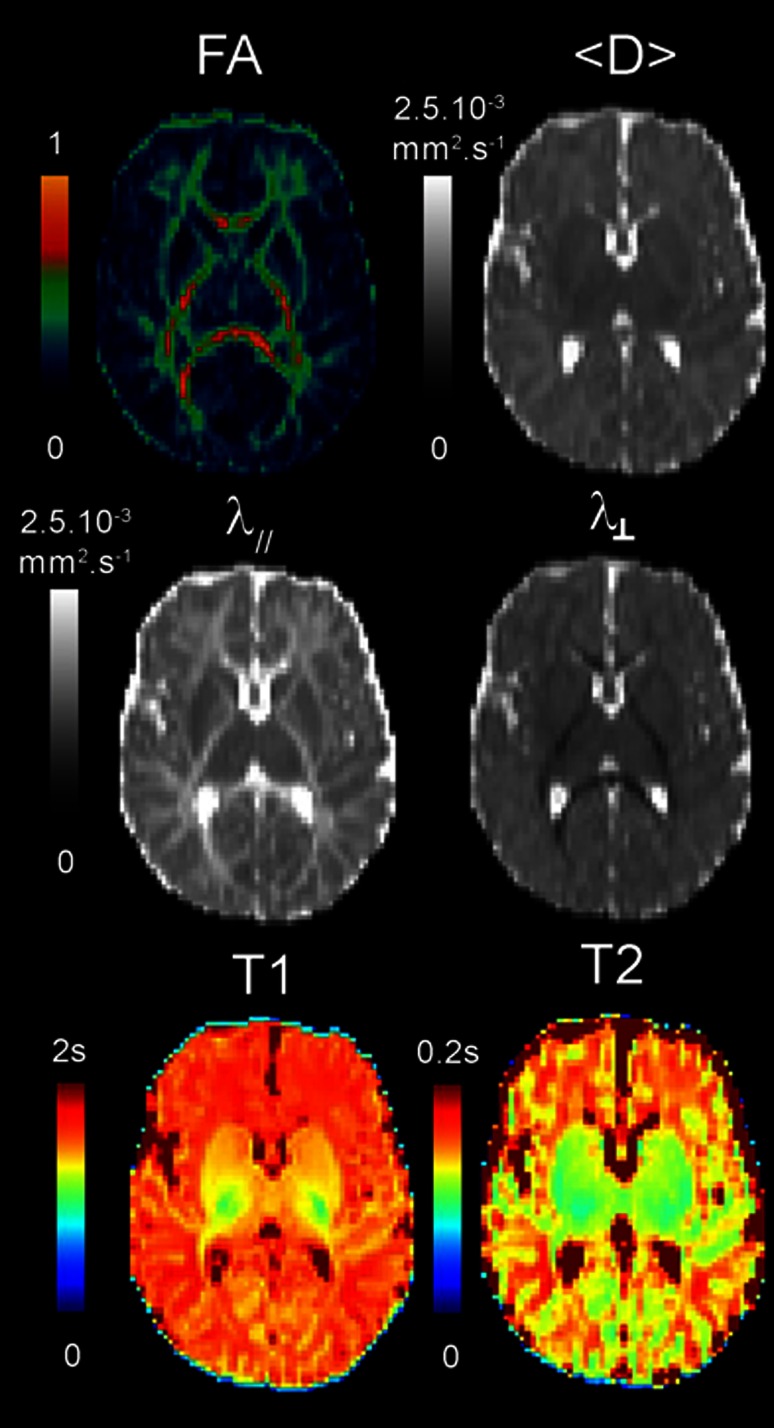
Quantitative maps of MRI parameters. Maps of DTI parameters and relaxation times are presented for a 6-week-old infant
